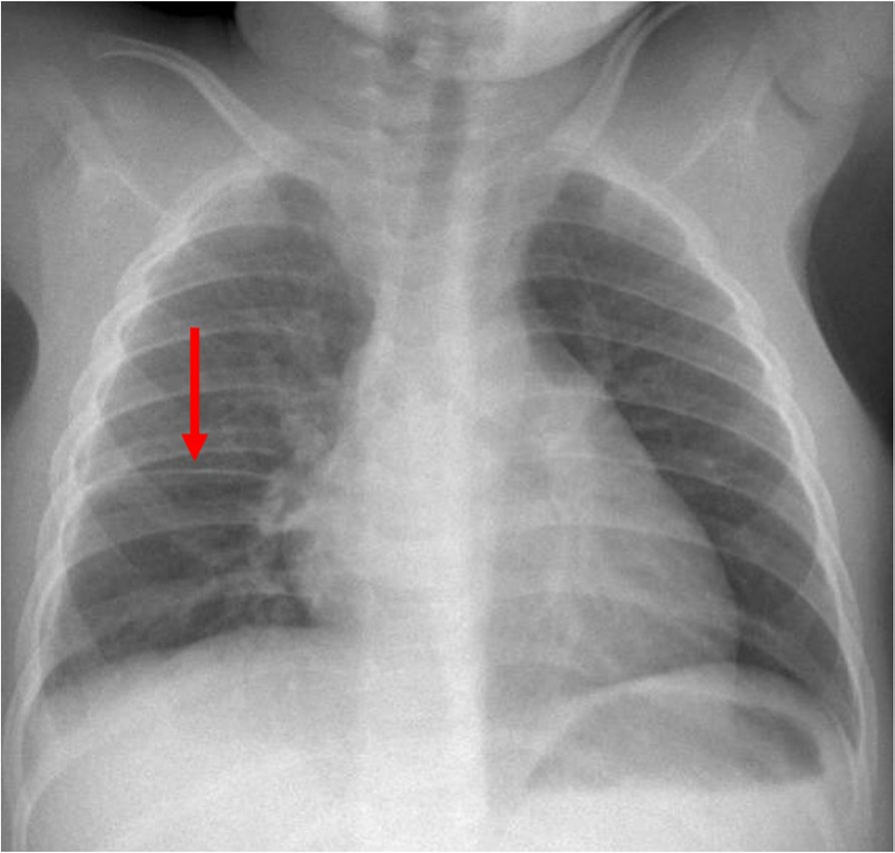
Normal chest X-ray 3 months after the spikelet aspiration, with only a slight pleural fissure thickening (red arrow)
